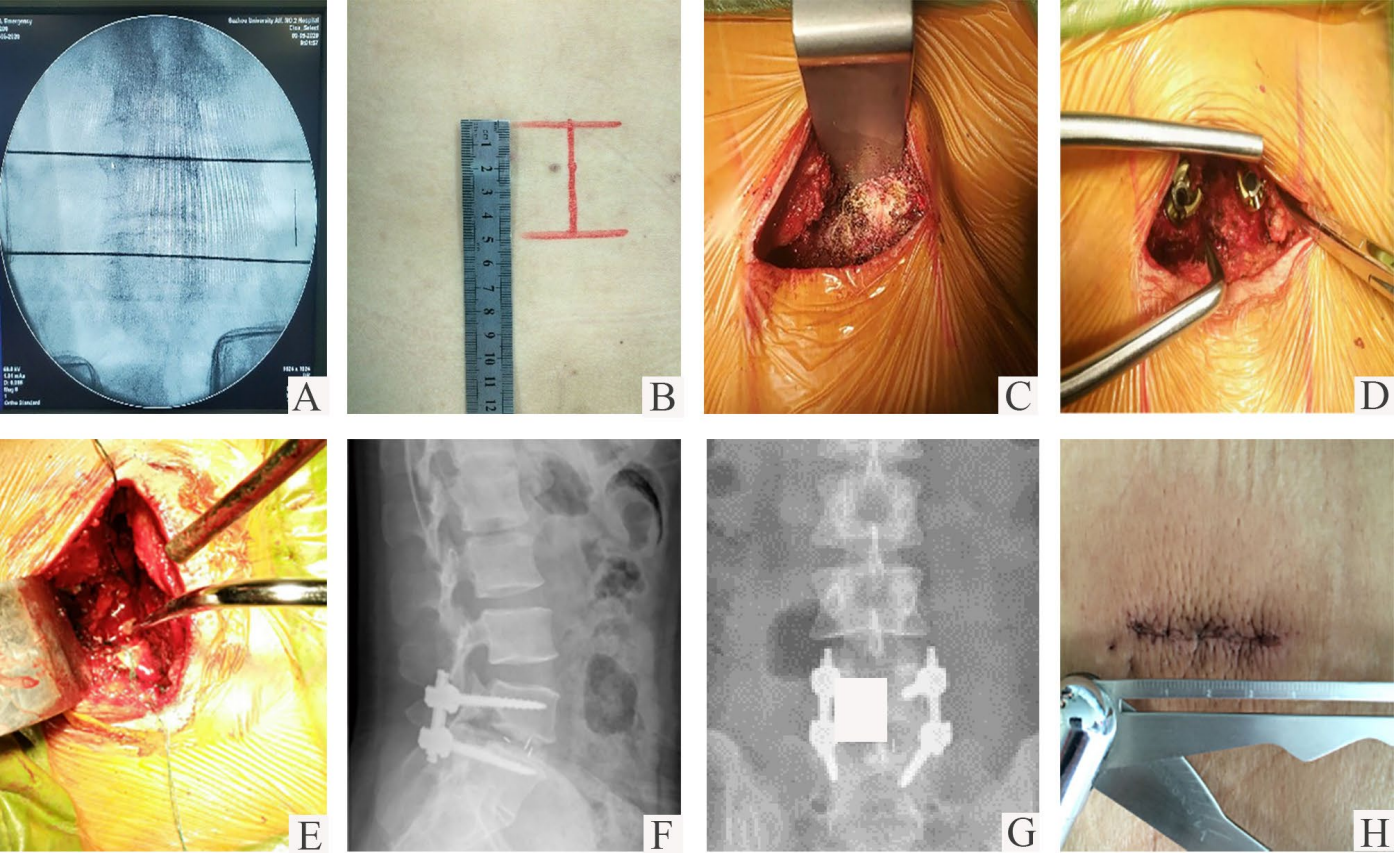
Surgical procedure of mMo-TLIF (modified Mini-Open Transforaminal Lumbar Interbody Fusion) via posterior midline incision. Preoperative localization of the surgical segment (a) and marked skin incisions (b); Along the Para-midline fat plane "point exposed" the pedicle screw entry area (c) and placement of the screw one by one (d); The "point exposure" for laminectomy with conventional lamina retractor (e); The anteroposterior and lateral X-ray results after operation (f, g); The appearance of midline 4.0 cm incision length (h)
